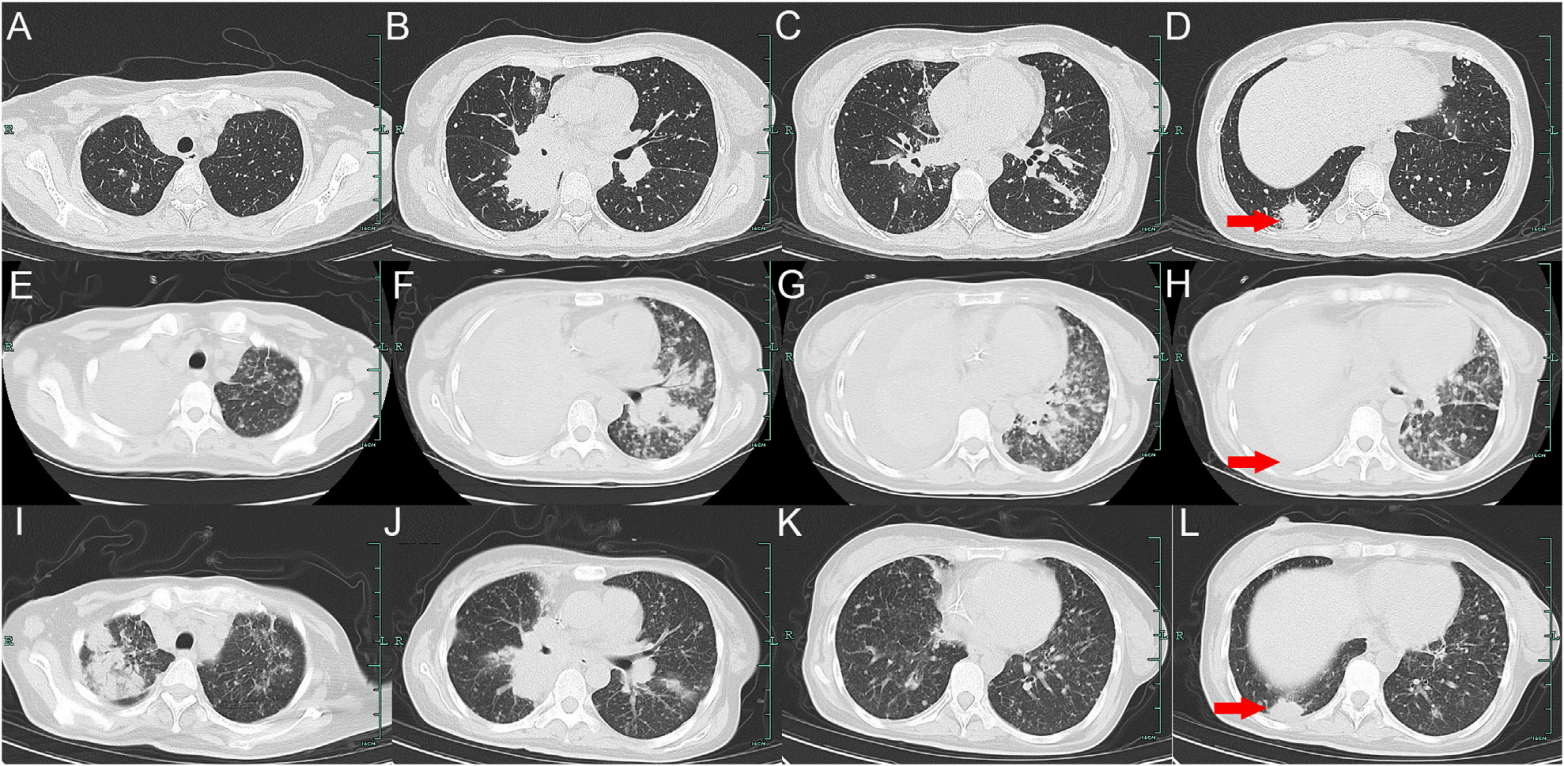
Variations in the primary lesions in the lung by chest CT scans during the treatment (red arrowheads). (A–D) were observed on 29 September 2023; (E–H) were observed on 1 November 2023; (I–L) were observed on 28 November 2023.
